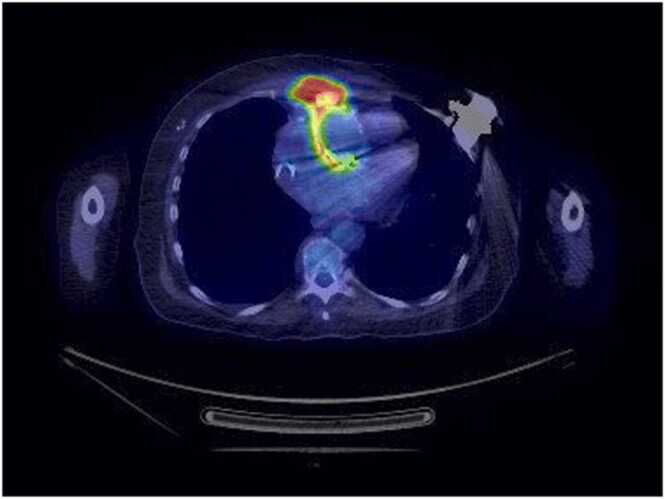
PET-CT image of the chest. PET-CT image demonstrating FDG uptake within the parasternal soft tissues with extension to the aortic root.
